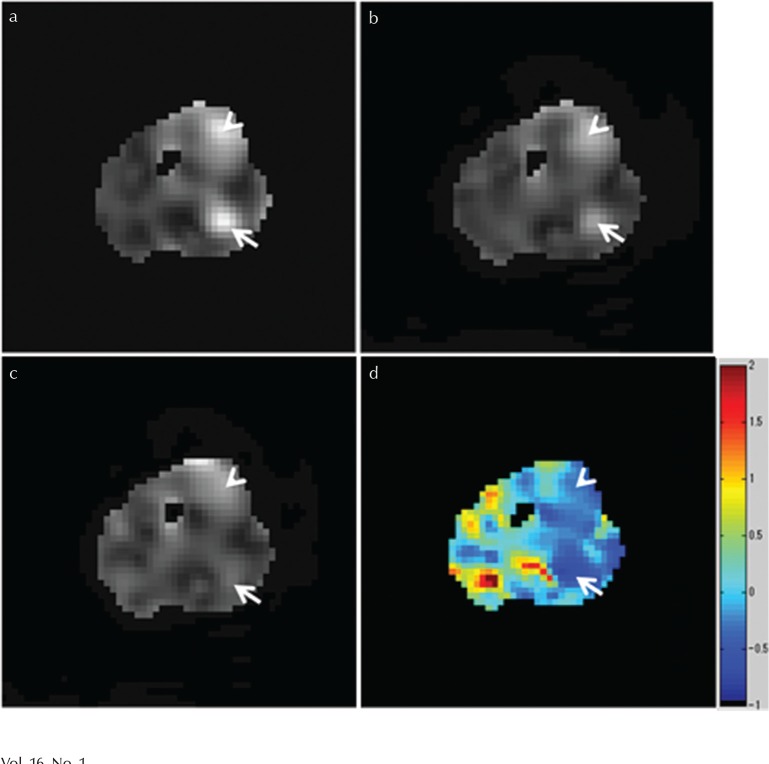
Axial MR images of a RA wrist. pCASL perfusion maps with PLD times of (a) 1025, (b) 1525, (c) 2025 ms are calculated by subtracting an image without blood preparation from that with blood preparation. (d) The BFPV map is presented as a color-coded image according to the BFPV values. The BFPV values of the radial artery (arrow) shows negative and smaller than those of pannus (arrow head). RA, rheumatoid arthritis; pCASL, pseudo-continuous arterial spin labeling, PLD, post-labeling delay, BFPV, blood-flow pattern variation.
